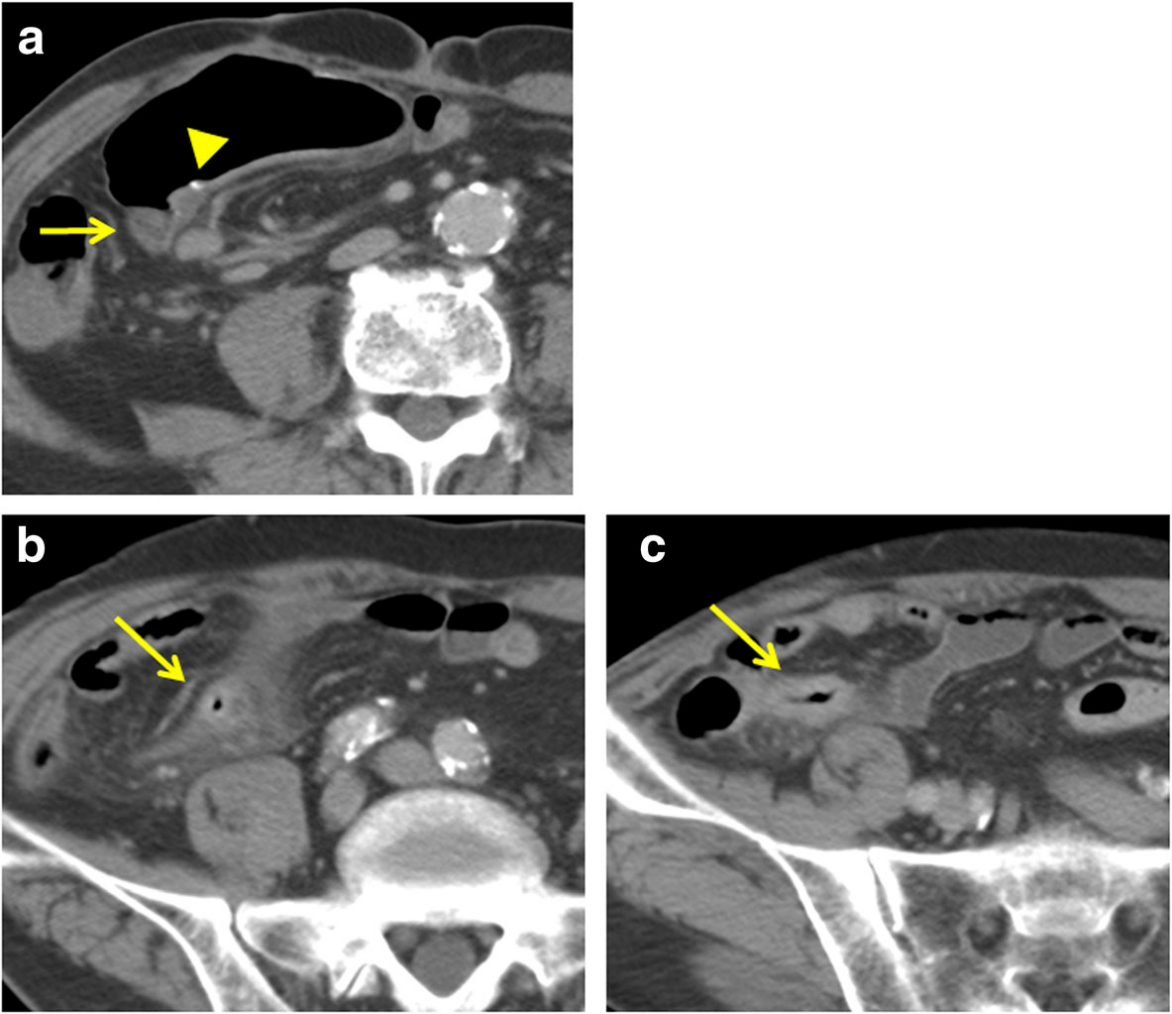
a Computed tomography (CT) scan of the small intestine, revealing diameter change on the distal side of the anastomotic site (arrow). The triangle indicates a staple of the functional end-to-end anastomosis. b, c Wall thickening and inflammation of the mesentery were observed at the stenotic site (arrow)
